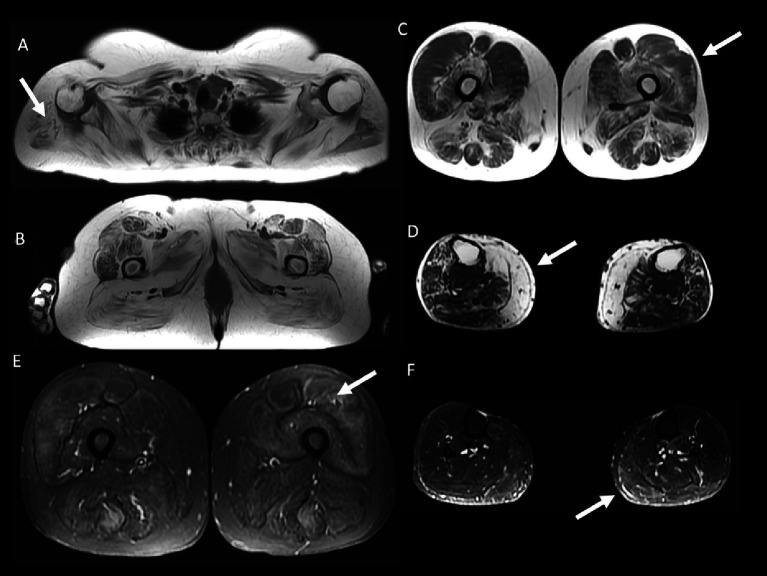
59 year-old female with inflammatory myopathy with mitochondrial pathology (IM-Mito). Axial fast spin-echo T1-weighted Whole-Body Muscle Magnetic Resonance Imaging (WBMRI) (A–D) showing severe fatty infiltration in the shoulder girdle muscles (A), moderate-severe fatty infiltration in quadriceps, gluteus and adductors muscles (B), moderate fatty infiltration in quadriceps muscle with relatively spared rectus femoris muscle compared to other quadriceps muscles (C) and severe fatty infiltration in the medial gastrocnemius muscle (D). Axial STIR WBMRI shows quadriceps muscle edema (E) and gastrocnemius muscle edema (F).
